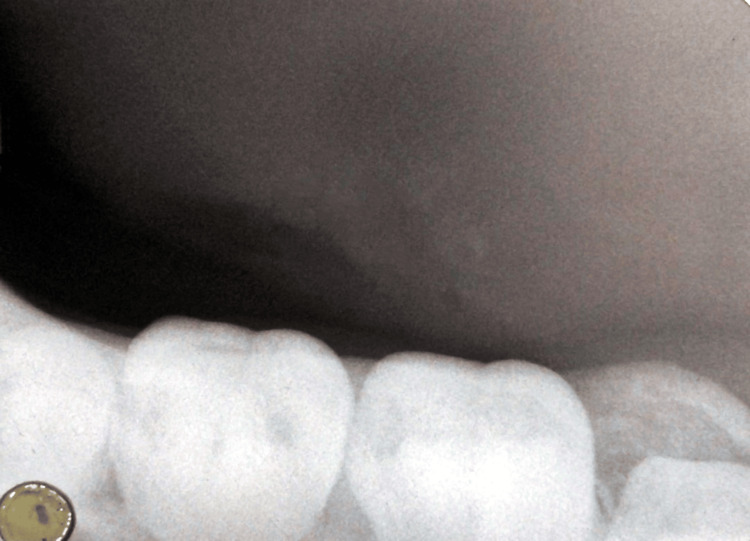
Occlusal radiograph obtained on the recall visit
